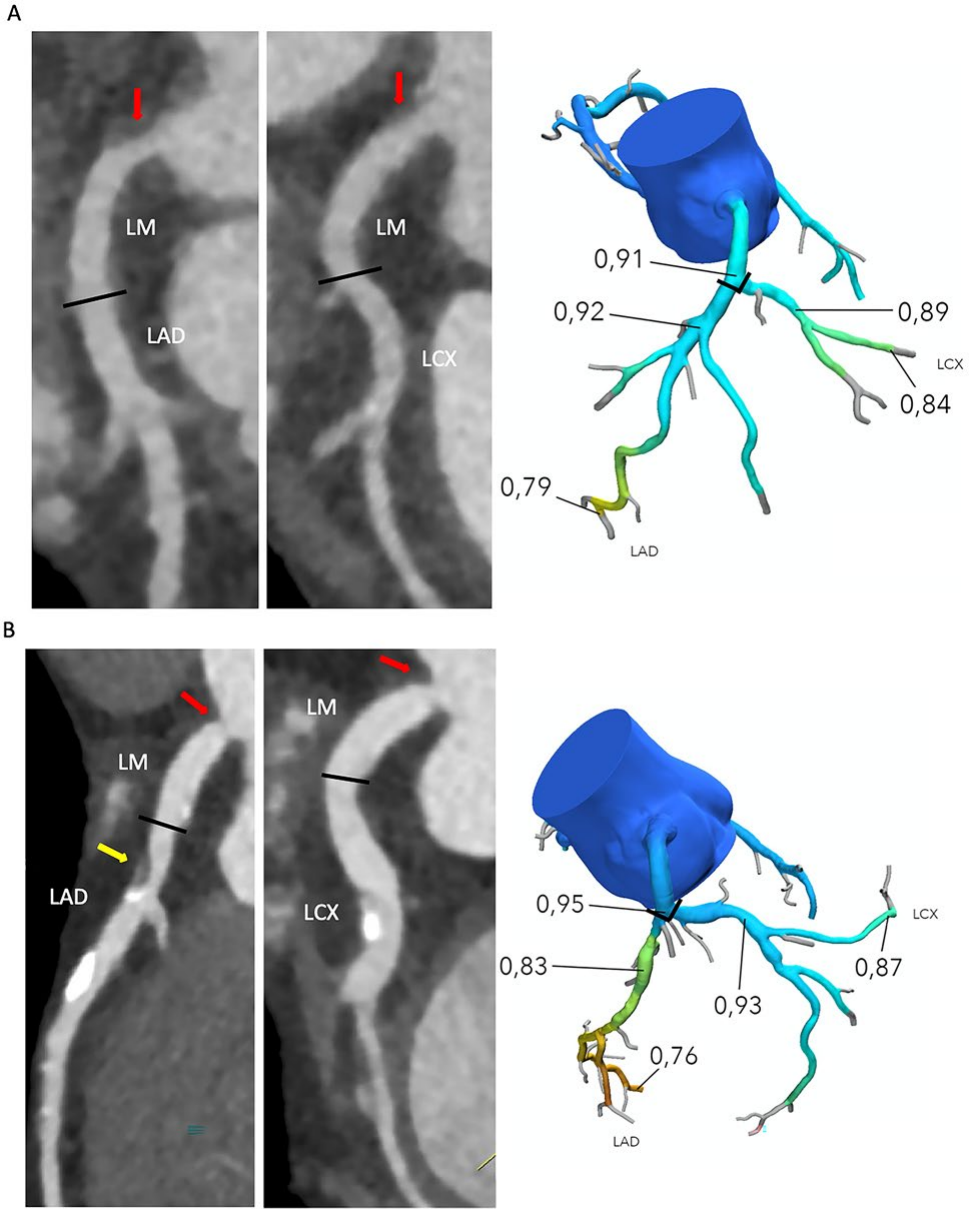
FFR_CT_ reading strategy. Examples of patients with simple (A) or complex (B) left main coronary artery disease (LMCAD). FFR_CT_ was registered 1. distally in the LM 2. in the proximal left anterior descending (LAD) and left circumflex artery (LCX) segments, and 3. distal segments. The first diagonal branch and first obtuse branch delineated proximal and non-proximal segments. Distal values were assessed in the most distal segments with lumen diameter > 1.8 mm. In patients with simple LMCAD (A) FFR_CT_ was registered in all segments 1–3. In patients with complex LMCAD (B) downstream FFR_CT_ were registered only in non-stenotic arteries. Thus, FFR_CT_ values in example B were registered only in the distal LM, and proximal and mid LCX segments. Left: Coronary CT angiography curved multiplanar reconstructions. Right: Three-dimensional FFR_CT_ model. The red arrows indicate the location of LMCAD. The yellow arrow denotes a proximal 60% diameter stenosis in the LAD
